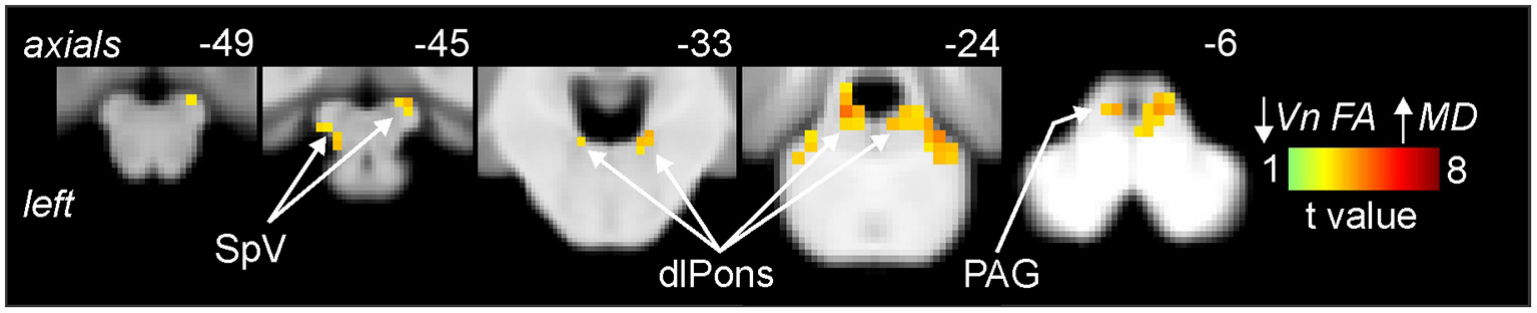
Areas of the brainstem in which reductions in trigeminal nerve fractional anisotropy (FA) were significantly correlated with increases in brainstem mean diffusivity (MD) assessed in migraine (*n* = 32) and control participants (*n* = 31). Significant clusters are overlaid onto a mean T1-weighted brainstem template image. Slice locations in Montreal Neurological Institute (MNI) space are indicated to the top right of each slice. Note that MD was significantly correlated to nerve FA in the brainstem regions of the spinal trigeminal nucleus (SpV), dorsolateral pons (dlPons) and the periaqueductal gray matter (PAG).
